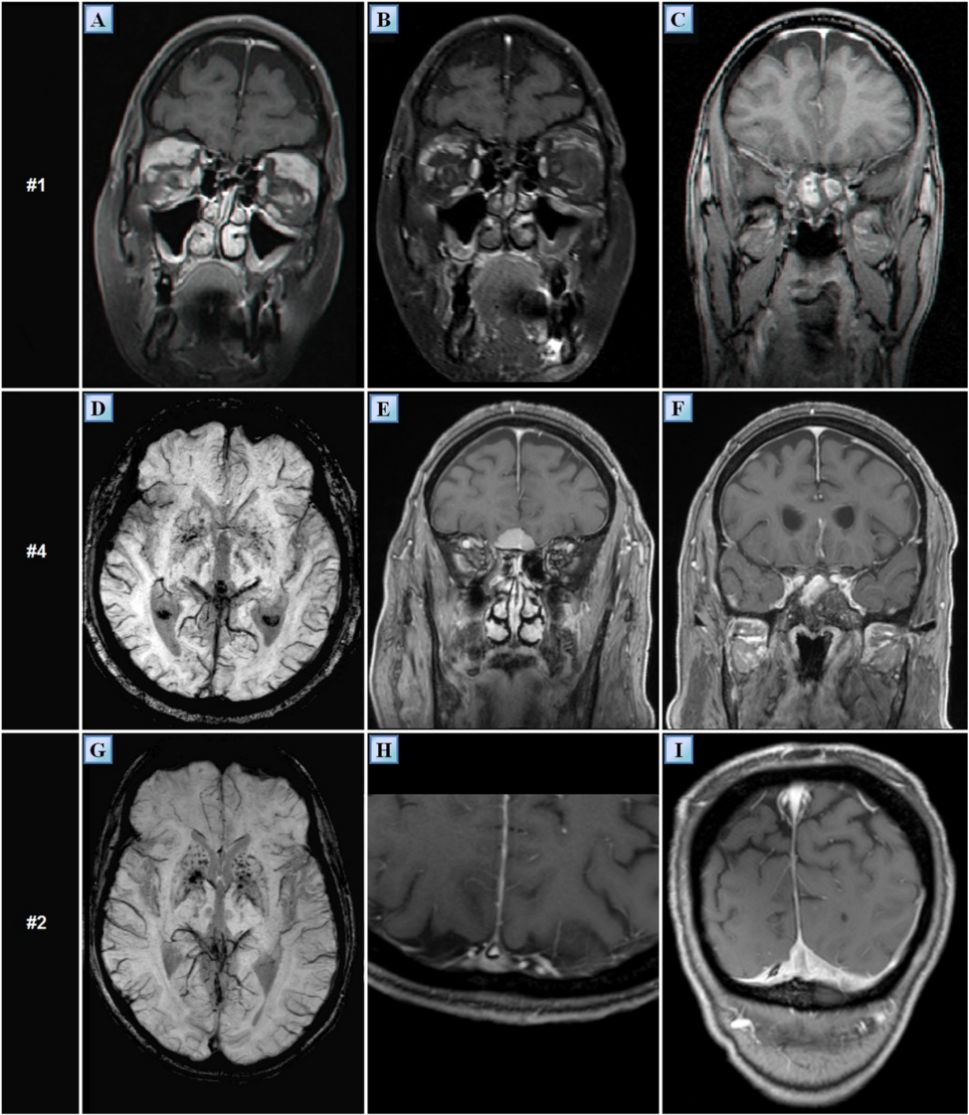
Various intracranial MRI findings of patients #1, #2 and #4. Coronal T1 weighted, gadolinium enhanced MR images of the retro-bulbar regions of patient #1 one year following diagnosis (A) and 4.5 years after diagnosis (B). The former (A) demonstrates bilateral nodular masses located at the superior lateral aspect of the retro-orbit. These masses involve both the lacrimal glands and superior rectus muscles and undergo heterogeneous enhancement following gadolinium administration. The latter (B) demonstrates a marked reduction in the size of these lesions, presumably due to treatment with interferon-α. (C) Coronal T1 weighted, gadolinium enhanced MR image of patient #1 showing mucosal thickening in the sphenoidal sinus as well as soft tissue fullness which undergoes enhancement following gadolinium administration. This finding is also apparent in patient #4 (F), who also exhibits an extra axial enhancing lesion situated in the vicinity of the planum sphenoidale (E). In SWI sequence, both patients #4 (D) and #2 (G) exhibit multiple punctate hypointensities in the basal ganglia. These patterns are not typical for senile calcifications. Patient #2 also exhibits infiltrative enhancing tissue which narrows the transverse left sinus, adjacent to the falx and tentorium (I). The same tissue is seen displacing the superior sagittal sinus (H). MR, magnetic resonance; SWI, susceptibility weighted imaging.
